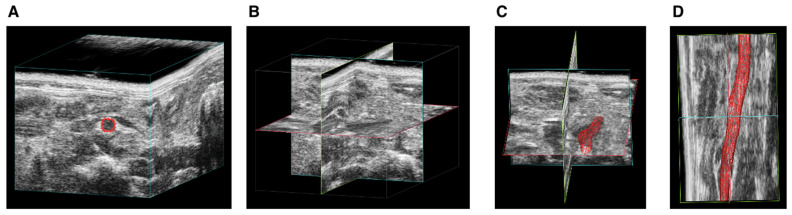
Steps of ultrasound (USS) three-dimensional lumen volume measurement (3DLV) reconstruction of the suprarenal and infrarenal aorta. (A) Cube view of the infrarenal aorta, (B) cross view, (C) surface view, and (D) overlay view.
